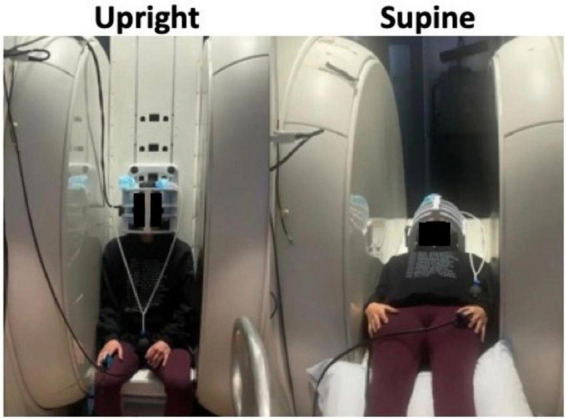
Image of subject scanned in an upright MRI scanner showing how it is possible to shift the position between upright (left) and supine (right) within the MRI scanner itself. Adapted with permission from Muccio et al. (2021), *Fluids Barriers CNS*.
